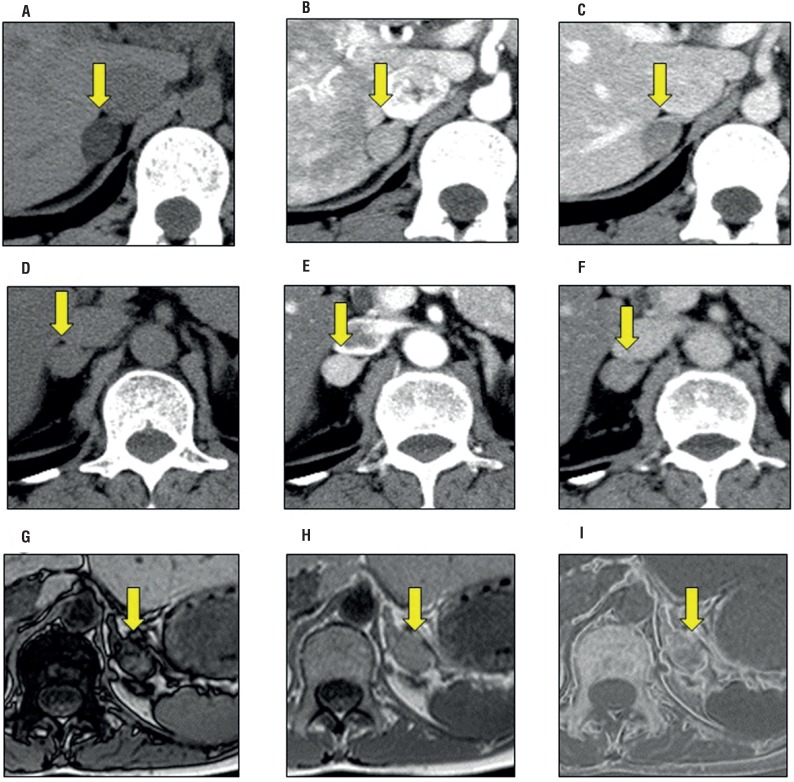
Radiological images of adrenal adenoma. a-c: Lipid rich typical adrenal adenoma. (a) Unenhanced CT, (b) Early phase on contrast enhanced CT, (c) Delayed phase on contrast enhanced CT. d-f: Lipid poor adrenal adenoma. (d) Unenhanced CT, (e) Early phase on contrast enhanced CT, (f) Delayed phase on contrast enhanced CT. g-i: Lipid poor adrenal adenoma. (g) Out-of-phase MR image, (h) in-phase MR image, (i) Chemical shift subtraction MR image. Arrows indicate the adrenal tumors.
